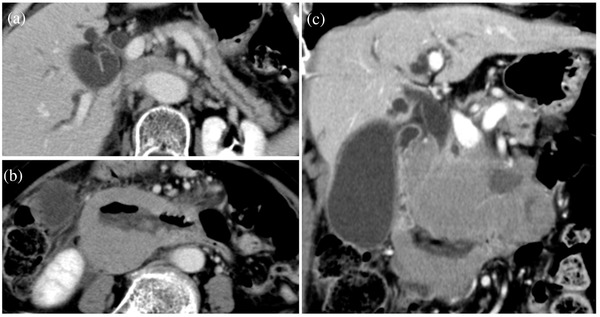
Computed tomography images: Abdominal computed tomography images of transverse sections (a,b) and coronal section (c) reveal a mass in the second portion of the duodenum. Duodenal stenosis, pancreatic duct dilatation, and bile duct obstruction are present
